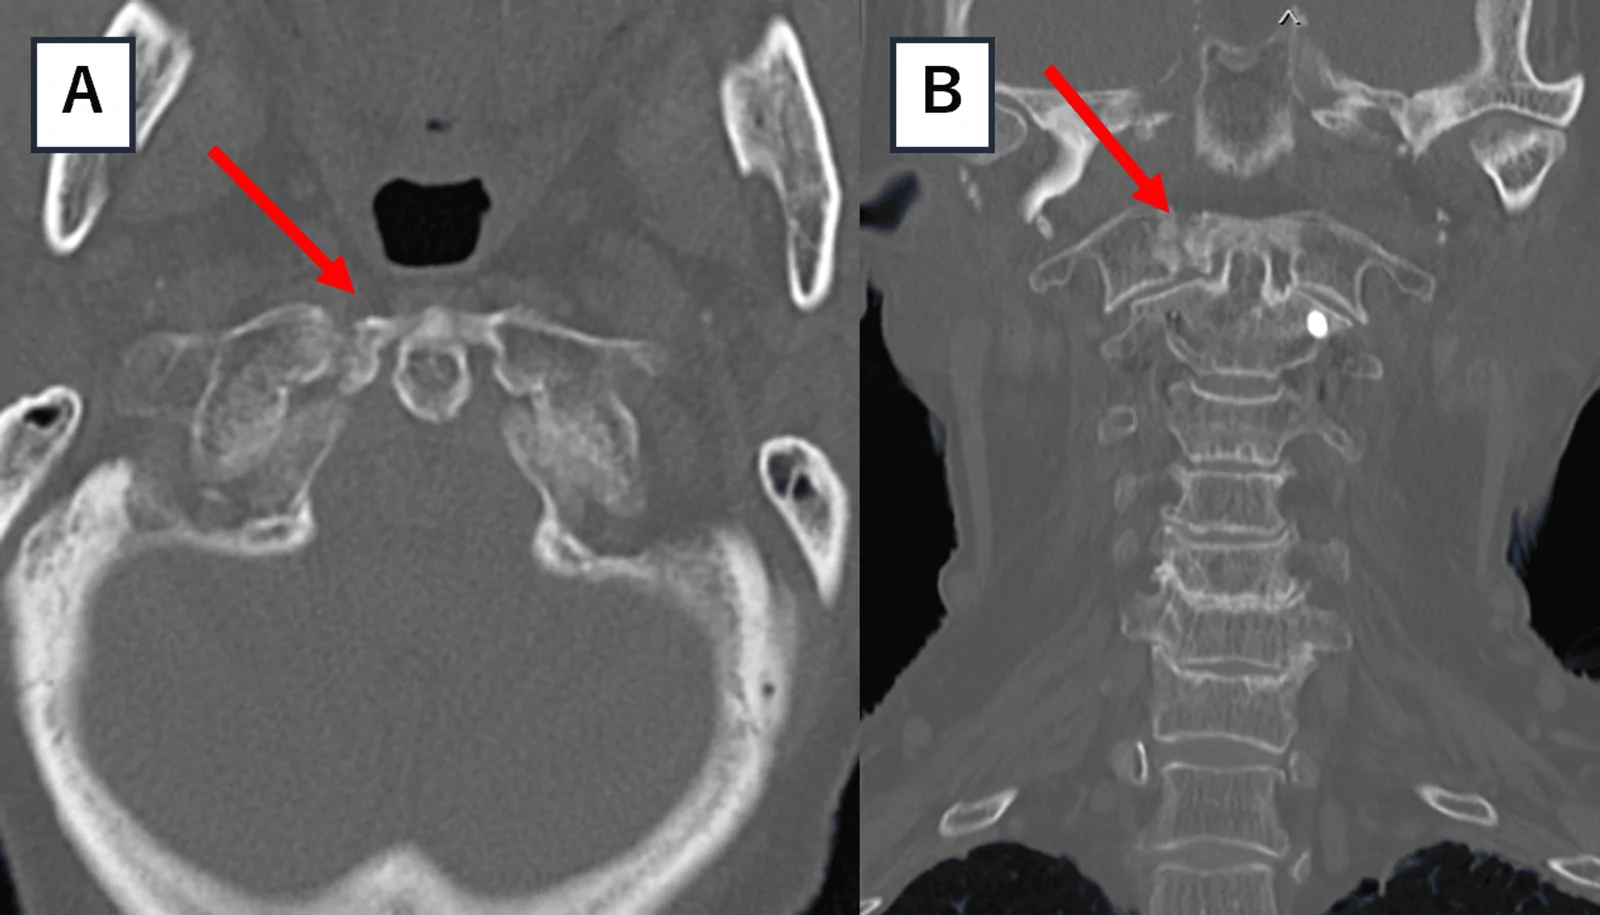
Follow-up imaging obtained nine months after occiput-to-C2 fixation (A) Axial CT image demonstrating progressive bone union. (B) Coronal CT image confirming maintained fixation and fusion progression
